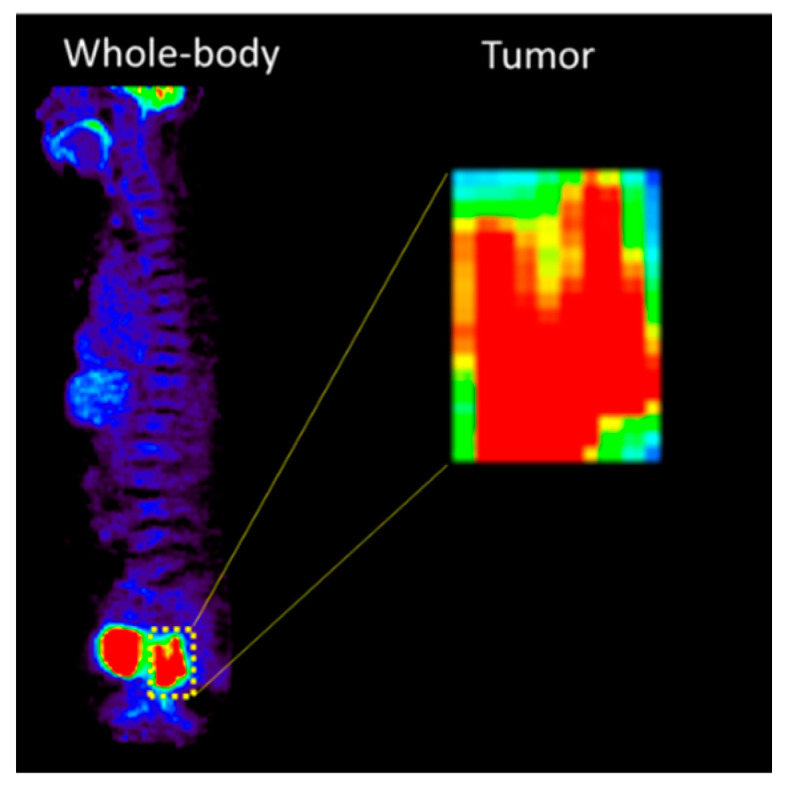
The corp process of rectal cancer region from ^18^F-FDG PET image.
